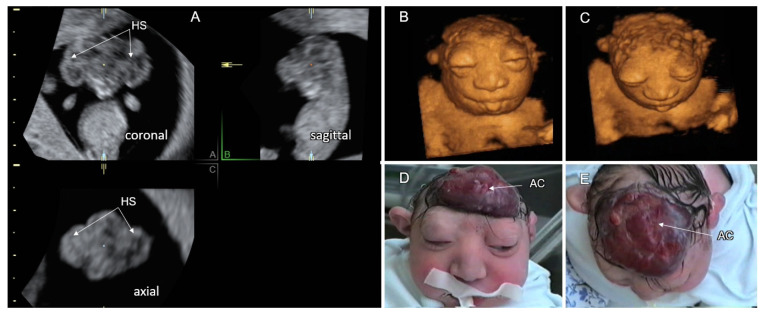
Progression from acrania to anencephaly. Legend: (A) A three-dimensional sonographic multiplanar reconstruction image demonstrating the coronal, mid-sagittal, and axial head planes of a fetus with acrania at 11 gestational weeks. Note the absent cranial vault and dysmorphic cerebral hemispheres (HS) floating in amniotic fluid above the base of the skull (exencephaly). (B,C) Three-dimensional sonographic surface render images of the fetal head at 27 gestational weeks. Note the flat, irregular area cerebrovasculosa (AC), a neural-vascular tissue remaining after traumatic destruction of the exposed cerebral hemispheres, the absent forehead, and protruding eyes. (D,E) Postpartum photographs corresponding to (B,C). The baby died 10 days after birth.
